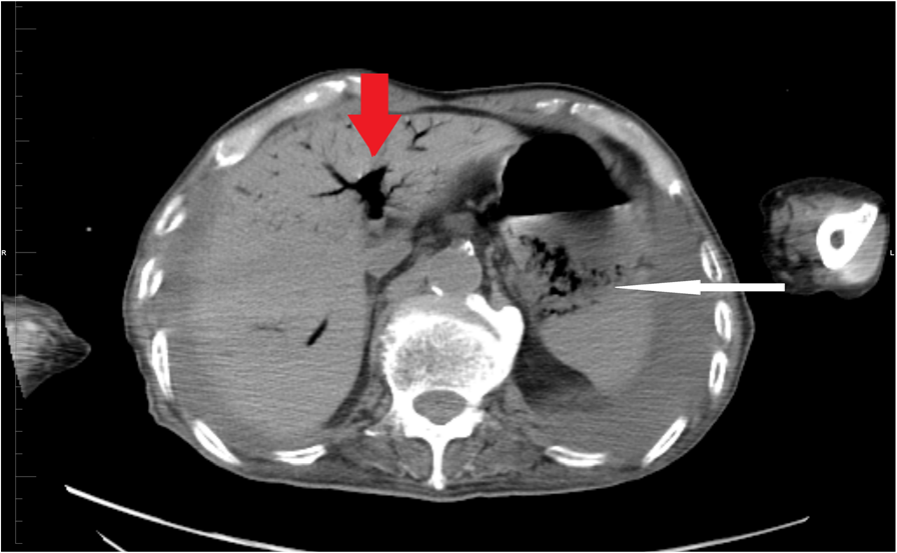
Computed tomography obtained on admission day showed hepatic portal venous gas (*thick arrow*) and air within the gastric wall (*thin arrow*)
